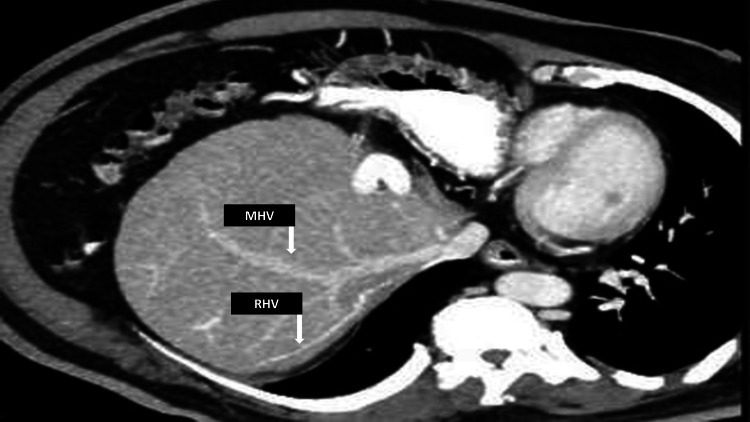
CT postcontrast axial view showing contrast opacified middle hepatic vein (MHV) and right hepatic vein (RHV).
